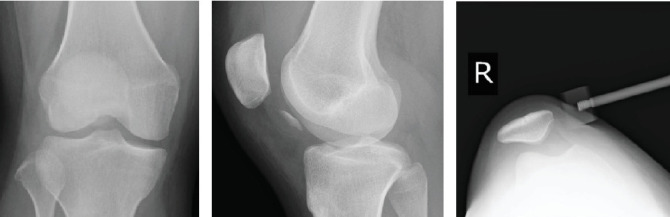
(a, b) X-ray images of a fracture fragment within the knee joint. (c) Patellar morphology is not abnormal, but the groove is slightly shallow and dislocation can be observed with external rotation stress of the knee and push to patellar.
